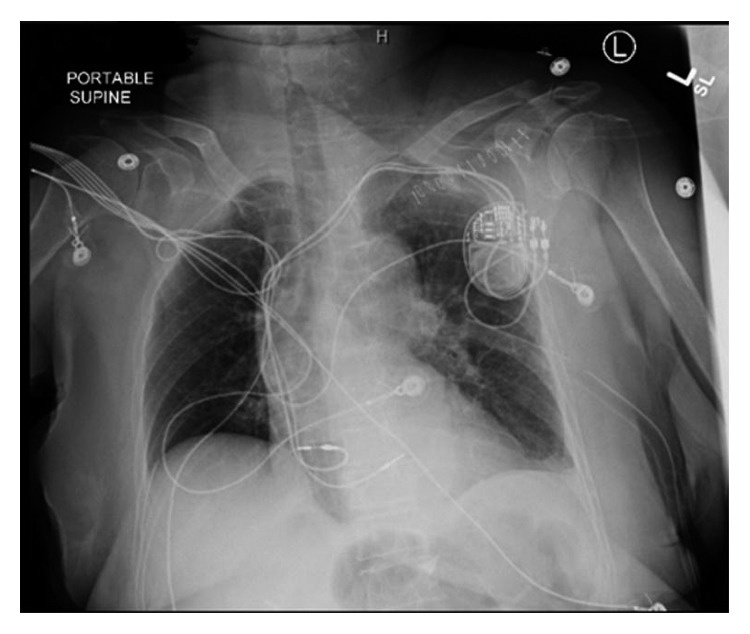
A chest X-ray showing the right ventricular lead residing at a normal position within the right ventricle after the revision.
